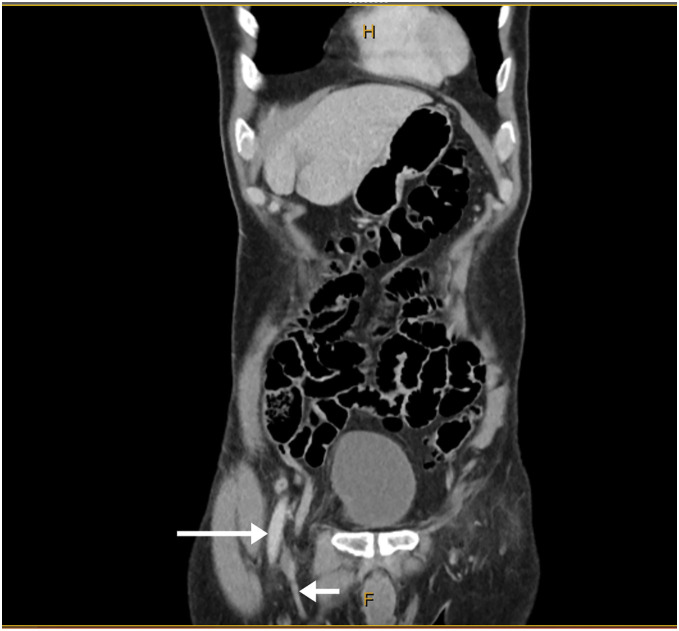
Coronal CT scan showing the appendix within the right femoral canal. The long arrow indicates the femoral artery and the short arrow indicates the femoral vein.
